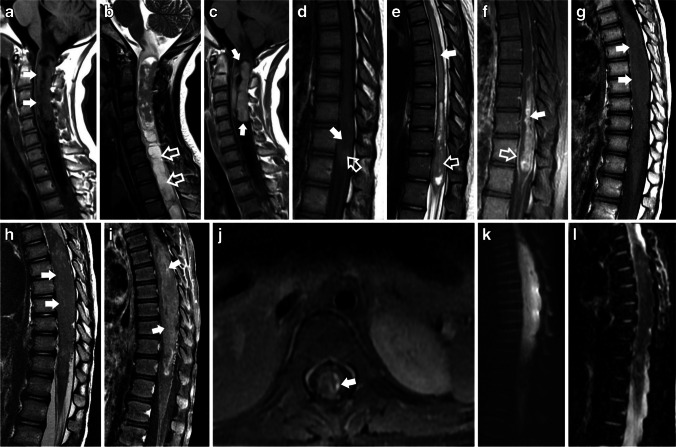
(a – k) Examples of the imaging features of high-grade vs. low-grade pediatric spinal gliomas. (a – c) Pilocytic astrocytoma in an 11-year-old girl, found incidentally. a) Sagittal T1 and b) T2 show a heterogeneous, solid and cystic/necrotic, expansile mass, extending from the cervico-medullary junction caudally to the C5- C6 level (solid white arrows in a), with an associated extensive caudal syrinx (hollow white arrows in b). c) Sagittal T1 FLAIR after contrast administration shows a sausage-shaped tumor with nodular/mass-like enhancement and well-defined/crisp borders (type 1a) (solid white arrows). (d – f) Diffuse pediatric-type High-grade glioma, H3-wildtype, and IDH-wildtype in a 13-year-old girl, who presented with a one-year history of back pain and headaches, and 3 weeks of acute worsening and loss of ability to walk. d) Sagittal T1-weighted MRI image highlighting an ill-defined, expansile lesion in the distal spinal cord. This lesion displays areas of slightly hyperintense signal (hollow white arrow), with a central zone which is isointense to the normal spinal cord (solid white arrow). In conjunction with the sagittal T2 FSE findings (e), the T1 hyperintense signal areas (hollow white arrows) are suggestive of hyperproteinaceous fluid. e) Sagittal T2 FSE shows the solid-cystic/necrotic morphology of the lesion (hollow white arrow), associated with extensive edema extending from T4 to T7 (solid white arrow). The mass is centered in the thoracic cord and extends from T8 to T12. f) Sagittal T1 with fat saturation after contrast administration, shows a nodular/mass-like enhancing pattern with ill-defined/shaggy borders (Type 1b) and central enhancement (solid white arrow). Leptomeningeal enhancement is also present (hollow white arrow). (g – l) Spinal ependymoma (WHO 3) in a 3-year-old boy with initial presentation of progressive lower limb weakness that resulted in paraplegia, over a 3-week course. g) Sagittal T1 and h) Sagittal T2 show a mostly T1/T2 isointense, expansile mass, extending from T4 to T12 (solid white arrows in g and h). i) Sagittal T1 with fat saturation after contrast administration demonstrates a nodular/mass-like enhancement and ill-defined/shaggy borders (Type 1b) (solid white arrows). j) Axial T1 post-contrast administration with fat saturation shows nodular leptomeningeal enhancement in the left side of the lesion (solid white arrow). k) DWI shows high signal intensity which corresponds to l) Low signal intensity on the ADC map, in keeping with restricted diffusion
